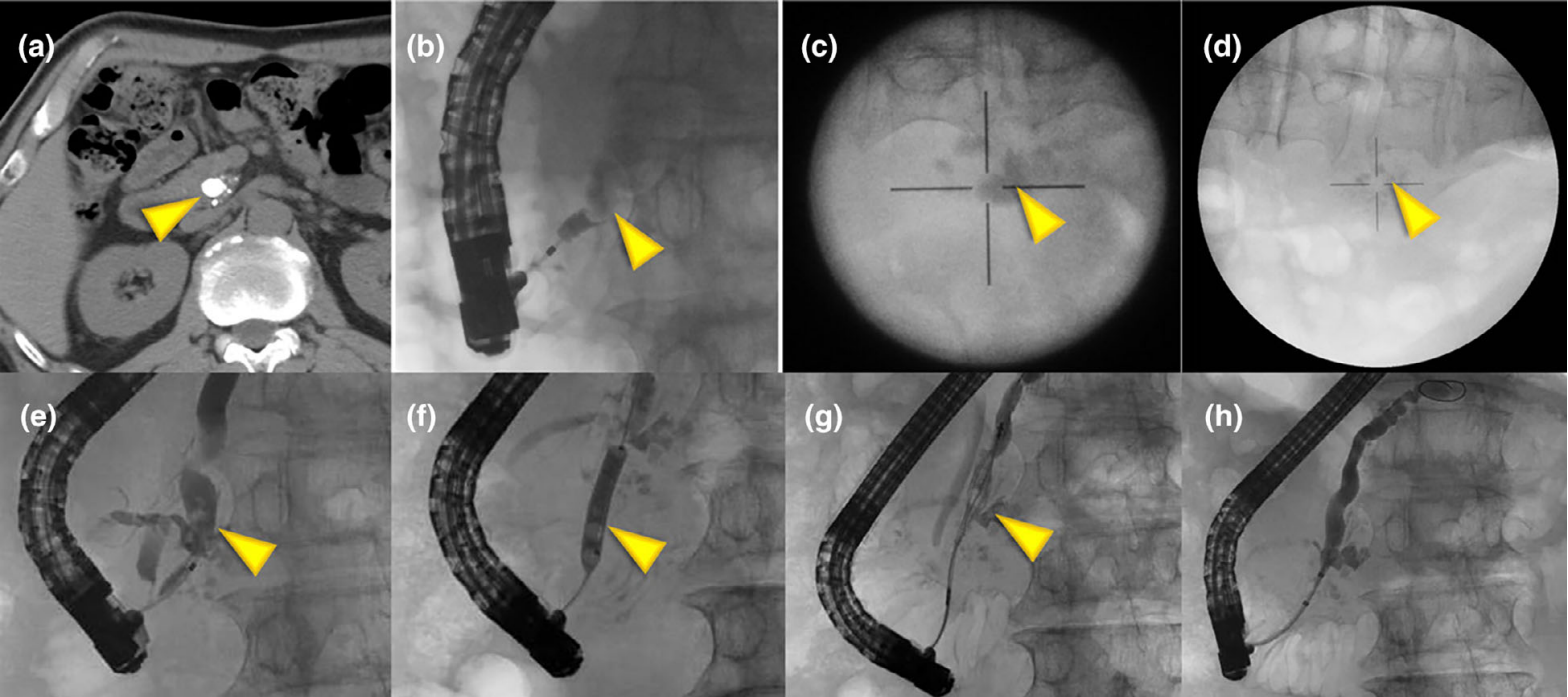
Extracorporeal shock wave lithotripsy (ESWL) and additional endoscopic stone removal. (a) Arrowhead shows symptomatic 12 mm stone at head of main pancreatic duct (MPD) (computed tomography value, 1137 Hounsfield units). (b) Endoscopic retrograde pancreatography showed impacted stone (arrowhead). (c, d) 26,000 shots of ESWL were performed and were effective (arrowhead). (e) Endoscopic retrograde cholangiopancreatography showing remaining stones in the head of MPD (arrowhead). (f) Using papillary balloon dilation. (g) Using basket forceps extraction (arrowhead), (h) Complete stone clearance.
